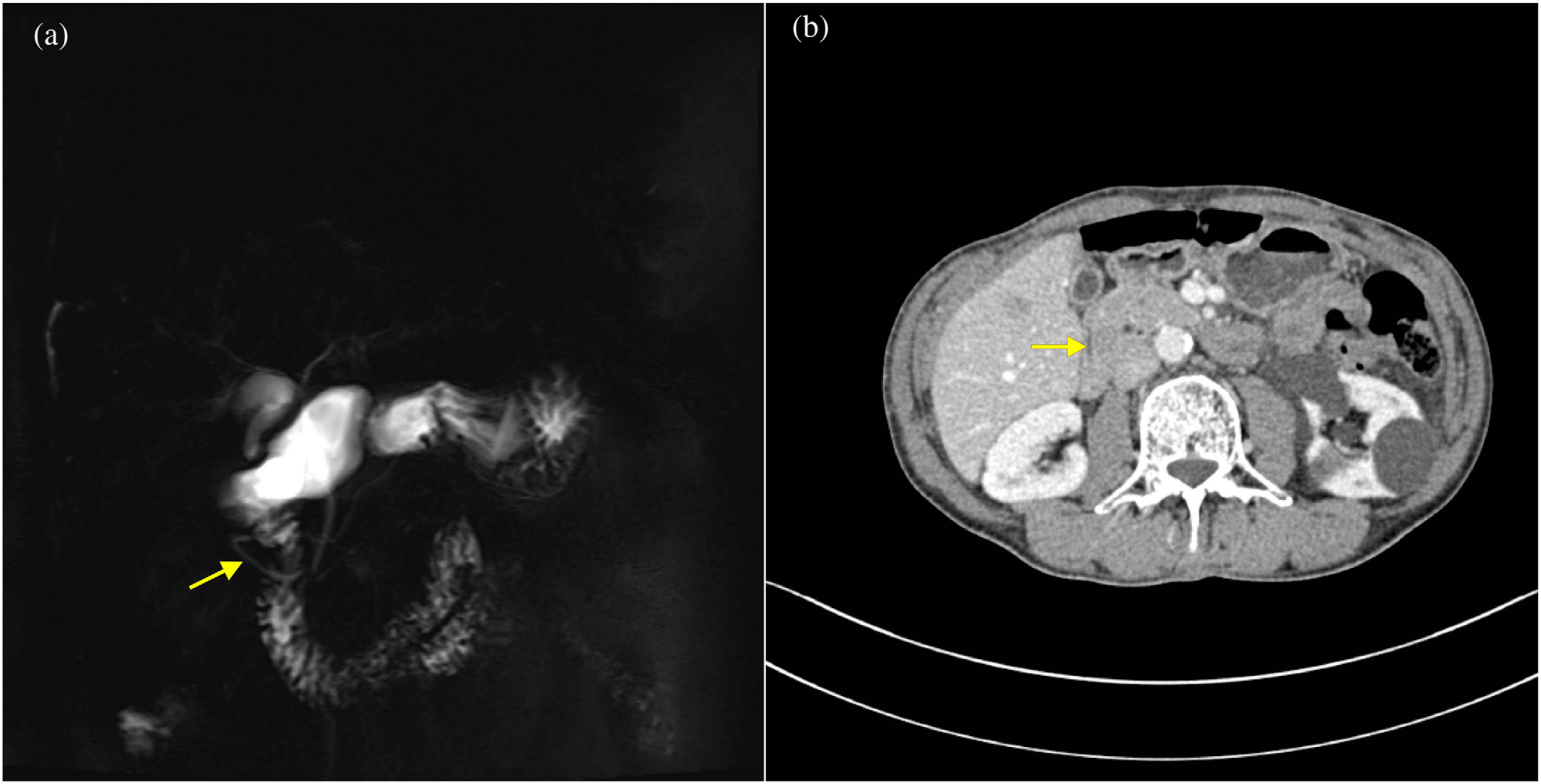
Magnetic resonance cholangiopancreatography (MRCP) image (a) shows the whole annular duct (arrow). Axial multiple detector spiral computed tomography (MDCT) (b) partially displays the annular duct in different slices of computed tomography (CT) imaging
